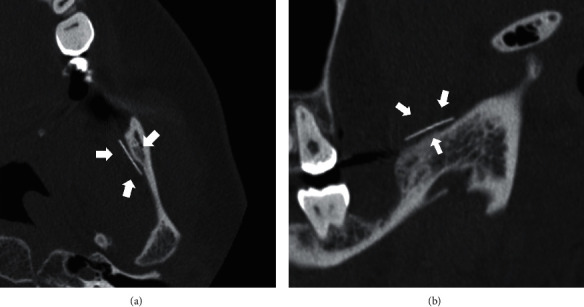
(a) Computed tomography (axial). The broken injection needle can be seen at the base of the left coronoid process (arrows). (b) Computed tomography (sagittal). The broken injection needle can be seen at the base of the left coronoid process (arrows).
